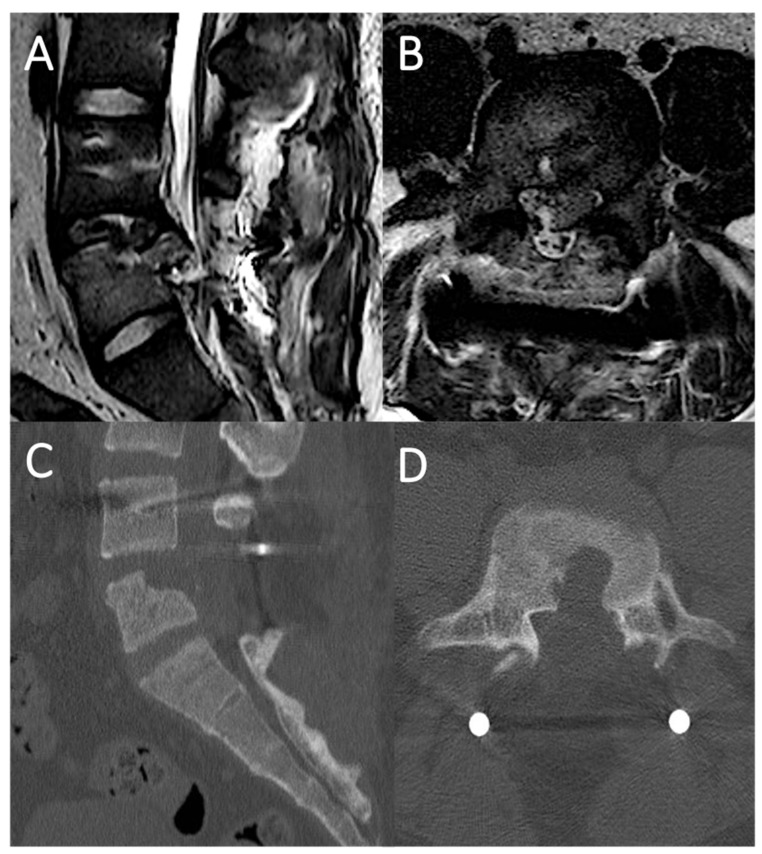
Lumbosacral spine MRI showing the L5 type A3 fracture with a single vertebral body fragment piercing the dural sac in sagittal (A) and axial view (B); Post-operative CT-scan after the revision surgery documenting the vertebral body fragment removal in sagittal (C) and axial view (D).
